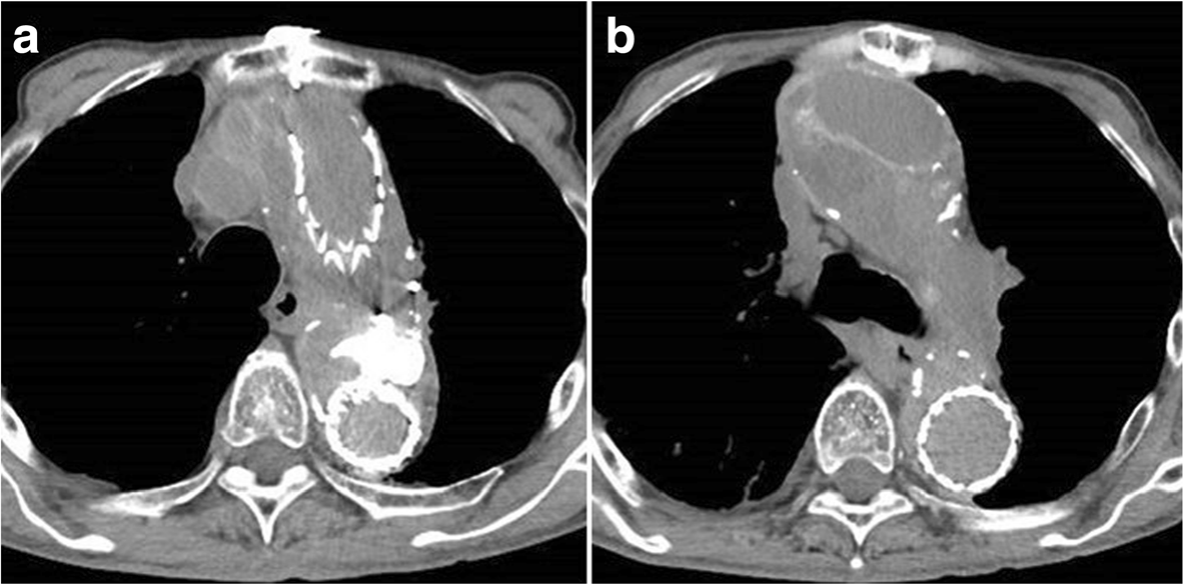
a and b. One year later, CT showed that the anastomotic pseudoaneurysms had disappeared, and the diameter of the aorta had also reduced
